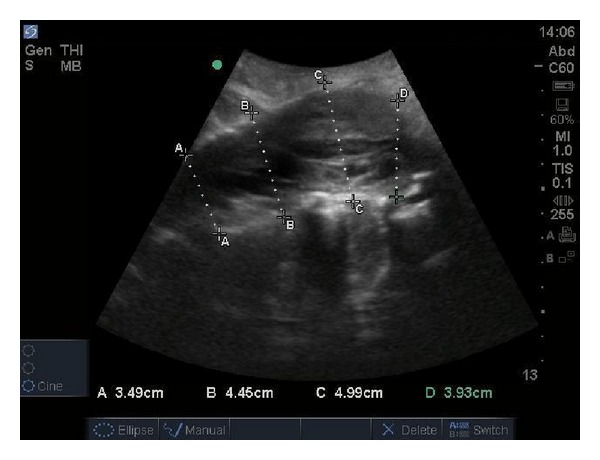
Abdominal ultrasonography demonstrating width measurements of aorta.
